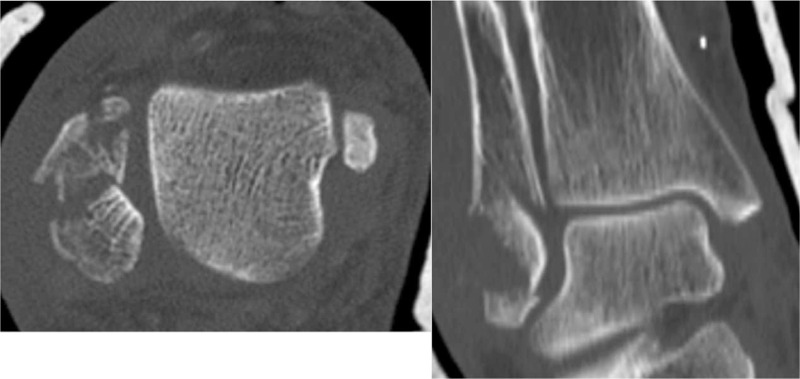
CT scan revealing the multifragmented nature of distal fibula.
